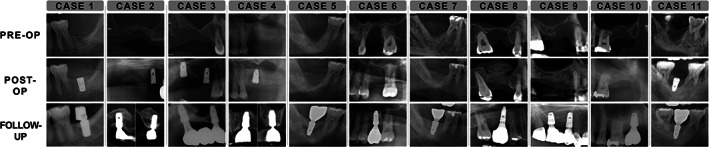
Radiographic findings for cases 1–11, including their preoperative, postoperative, and latest follow‐up data
